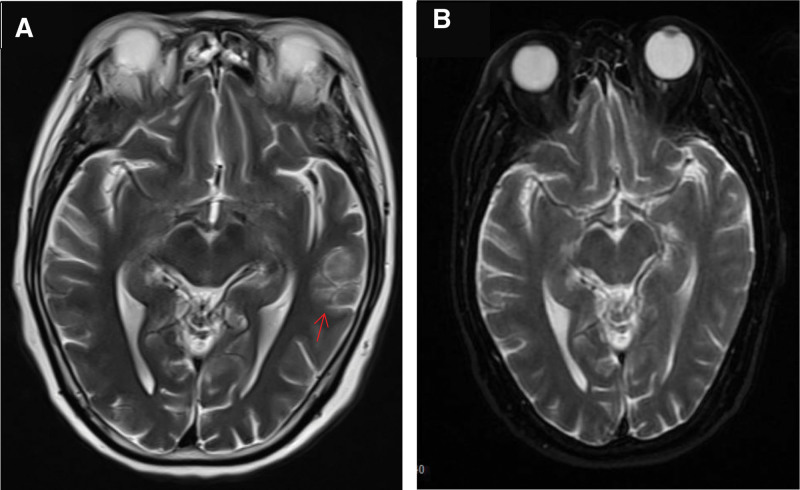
(A) T2-weighted MRI indicated a lesion with hyperintensity signal in left temporal lobe (red arrow). (B) T2-weighted MRI indicated the lesion in left temporal lobe disappeared after 10 d of treatment.
